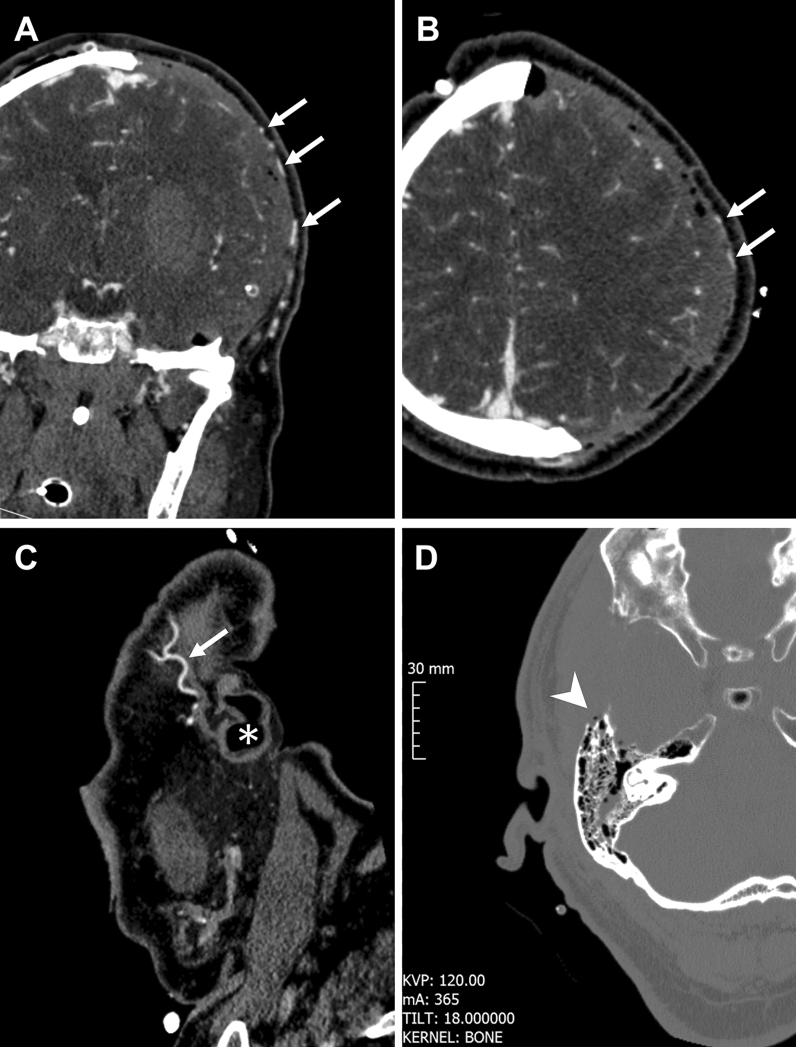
Preservation of the STA and accidental opening of the mastoid air cells. Representative postoperative CT angiography confirming preservation of the STA on (A) coronal, (B) axial and (C) sagittal plane. (D) shows breach of the bony air cells of the squamous segment of the temporal bone anterior to the mastoid. Arrows indicate the STA and * marks the external auditory canal. The arrowhead marks the opening of the bony air cells.
